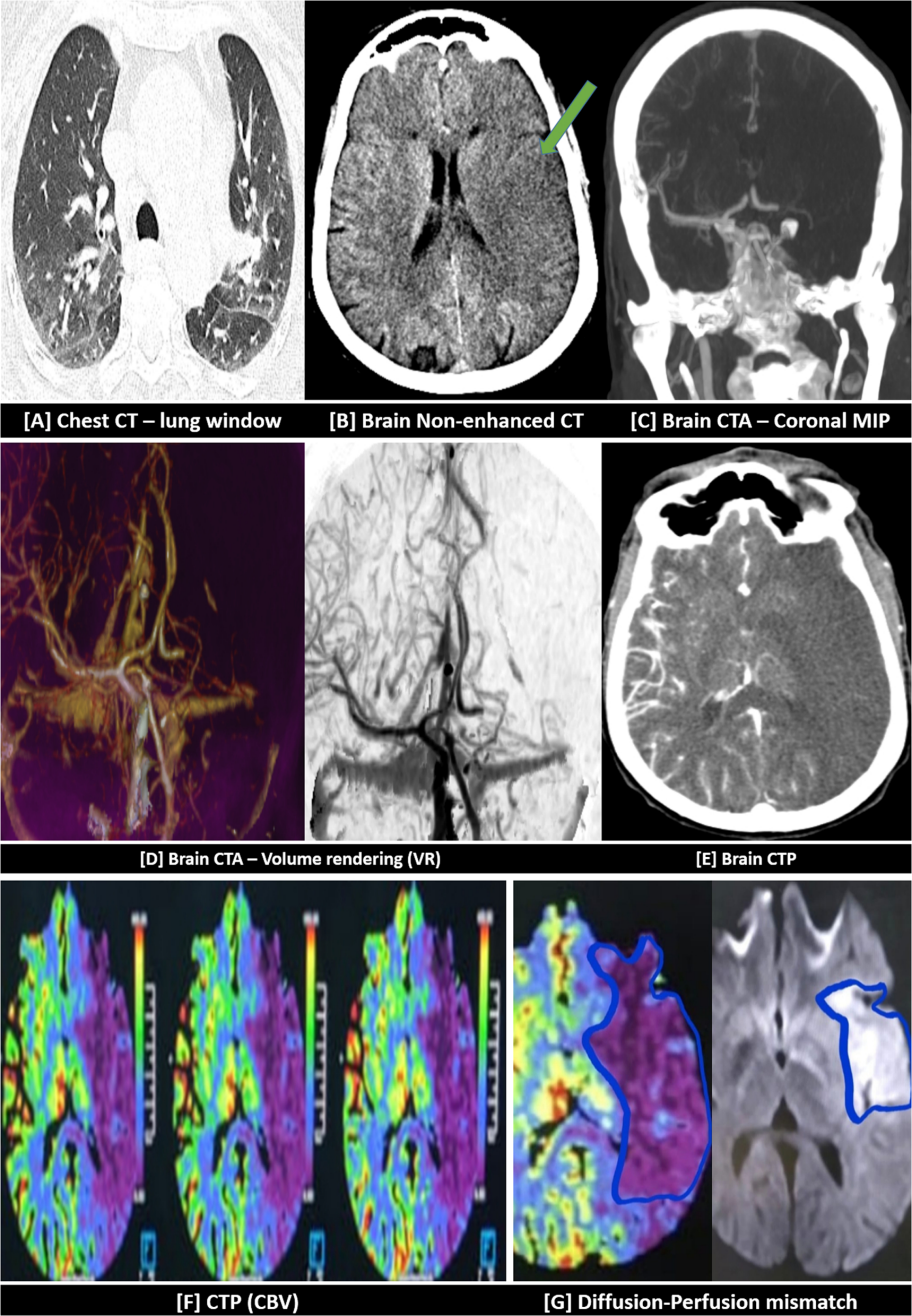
The above-mentioned patient in (Fig. 3) performed chest CT as well as brain CTA and CTP two hours later. [A] Chest CT image (lung window) revealed bilateral basal ill-defined ground-glass patches (suspicious for COVID-19 infection). [B] Initial non-contrast brain CT images revealed a large left frontotemporal, tempro-parietal, and tempro-occipital hypo-dense area (green arrow) implicating the cortical and subcortical white matter and extending to the left basal ganglia with effacement of the cortical sulci and left Sylvian fissure. CTA coronal MIP image [C] and volume rendering image [D] showed total occlusion and non-opacification of the intracranial segment of the left ICA and left MCA. Non-perfusion of this large area was noted in dynamic CT-perfusion [E, F]. An obvious diffusion-perfusion mismatch was noted denoting significant penumbra [G]. PCR test result was revealed to be positive for COVID-19
